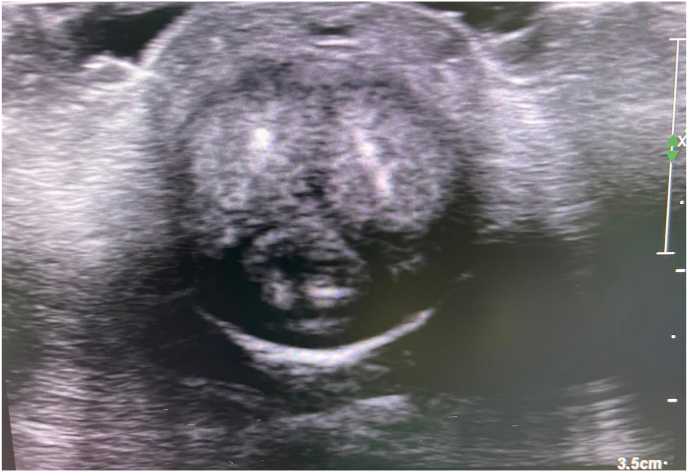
Penile duplex ultrasound demonstrated heavily calcification bilateral cavernosal penile arteries.
